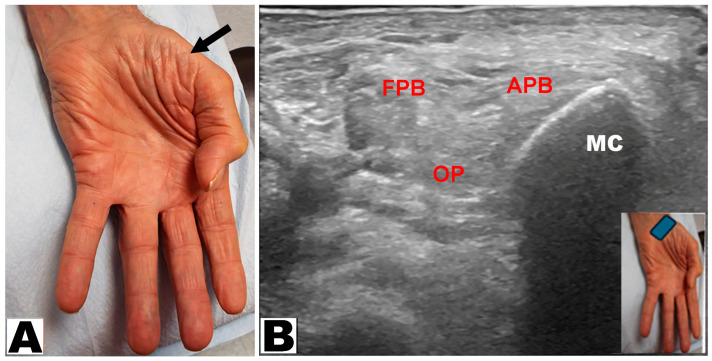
(A) Total atrophy of all thenar muscles of the hand (oblique arrow). (B) Total atrophy of the thenar muscles by ultrasound. APB: abductor pollicis brevis muscle. OP: opponens pollicis muscle. FPB: flexor pollicis brevis muscle. MC: metacarpal.
